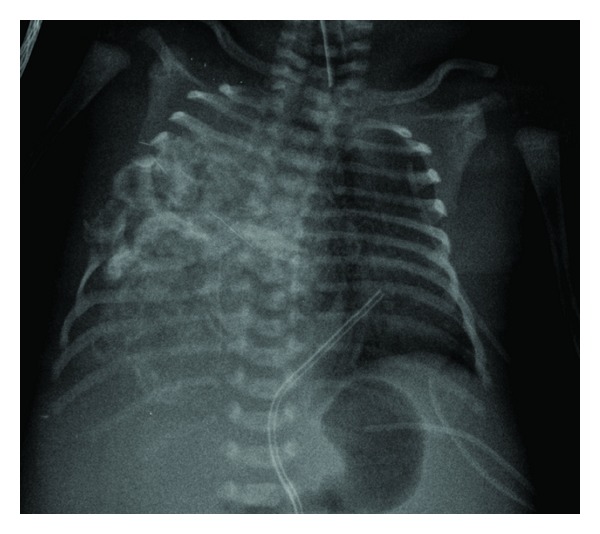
Chest X-ray showed right hemithorax opacification due to a large-sized mass with calcium density and destruction/erosion of the 4th–7th right costal arches, plus leftward tracheal deviation and centralized intestinal luminogram.
